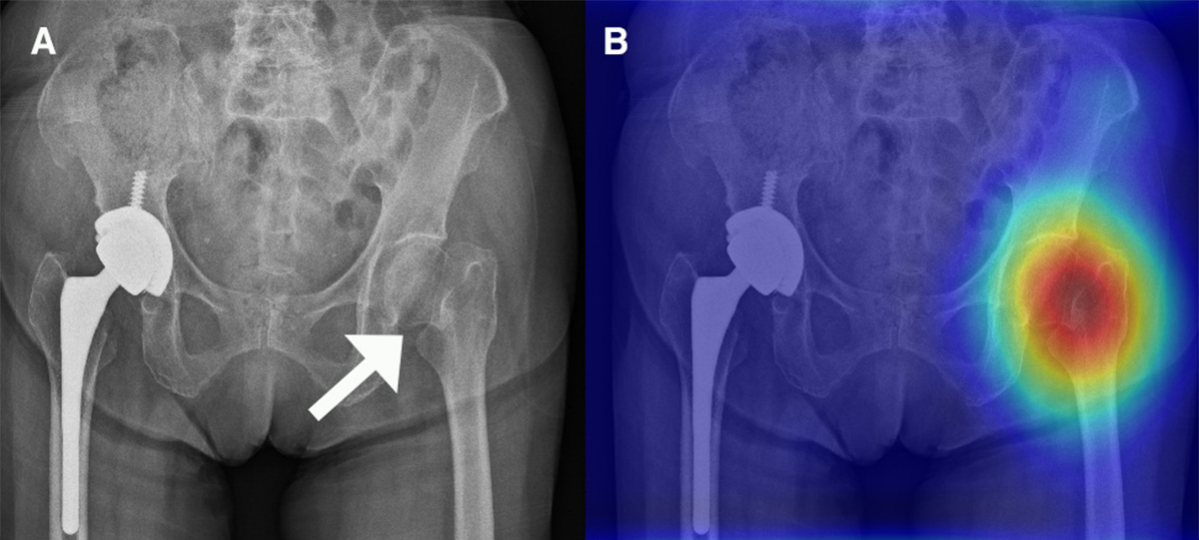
(A) Pelvic x-ray (PXR) with hip fracture. (B) PXR with left hip fracture with a human-algorithm integration–enhanced reference image.
